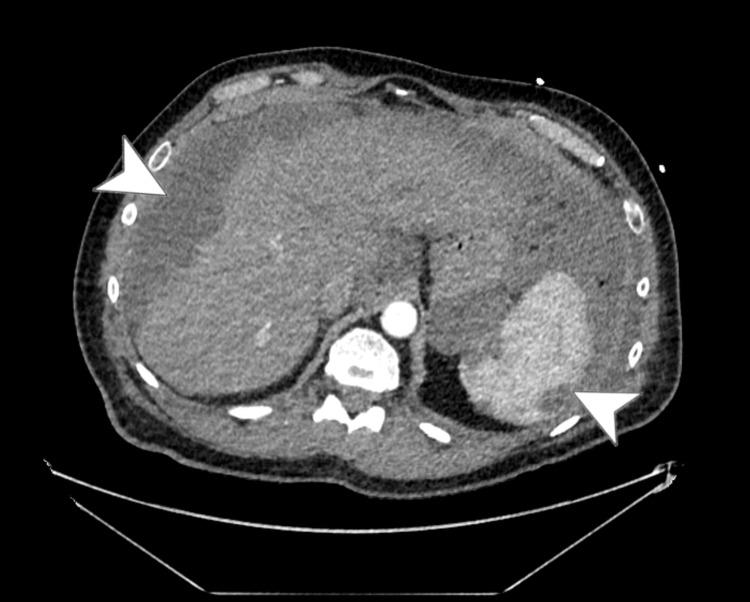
Axial CT view showing scalloping of the liver (left arrowhead) and spleen (right arrowhead)
